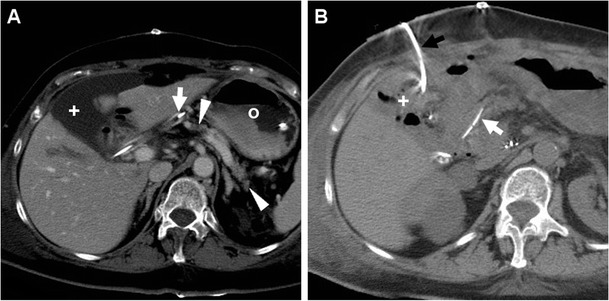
Bile collection found 4 days after PD for distal common bile duct (CBD) cholangiocarcinoma in an 83-year-old woman with biliary leakage from the laparotomic incision site. Contrast-enhanced CT (a) showed distended stomach (o) consistent with DGE, external drainage (*thick arrows*) in place, normal PR (*arrowheads*), and a non-encapsulated 10 × 5 cm water-attenuation collection (*) in the gallbladder fossa, which was treated with percutaneous drainage. Unenhanced follow-up CT (b) showed minimal residual fluid and air (+) in the site of the biloma
